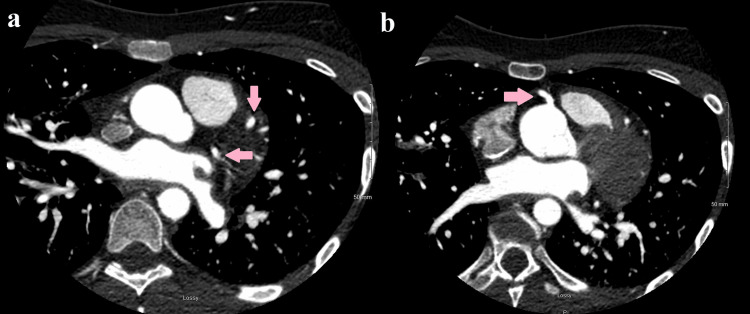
(a) Coronary computed tomography angiogram (CTA) demonstrating patent left-sided coronary arteries (pink arrows). (b) Coronary CTA demonstrating a patent right coronary artery (pink arrow).
